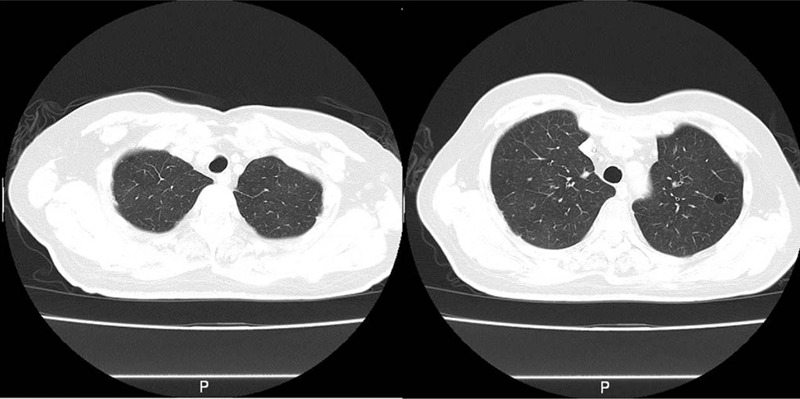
The initial stage of onset. Chest CT shows no obvious abnormalities. CT = computed tomography.
